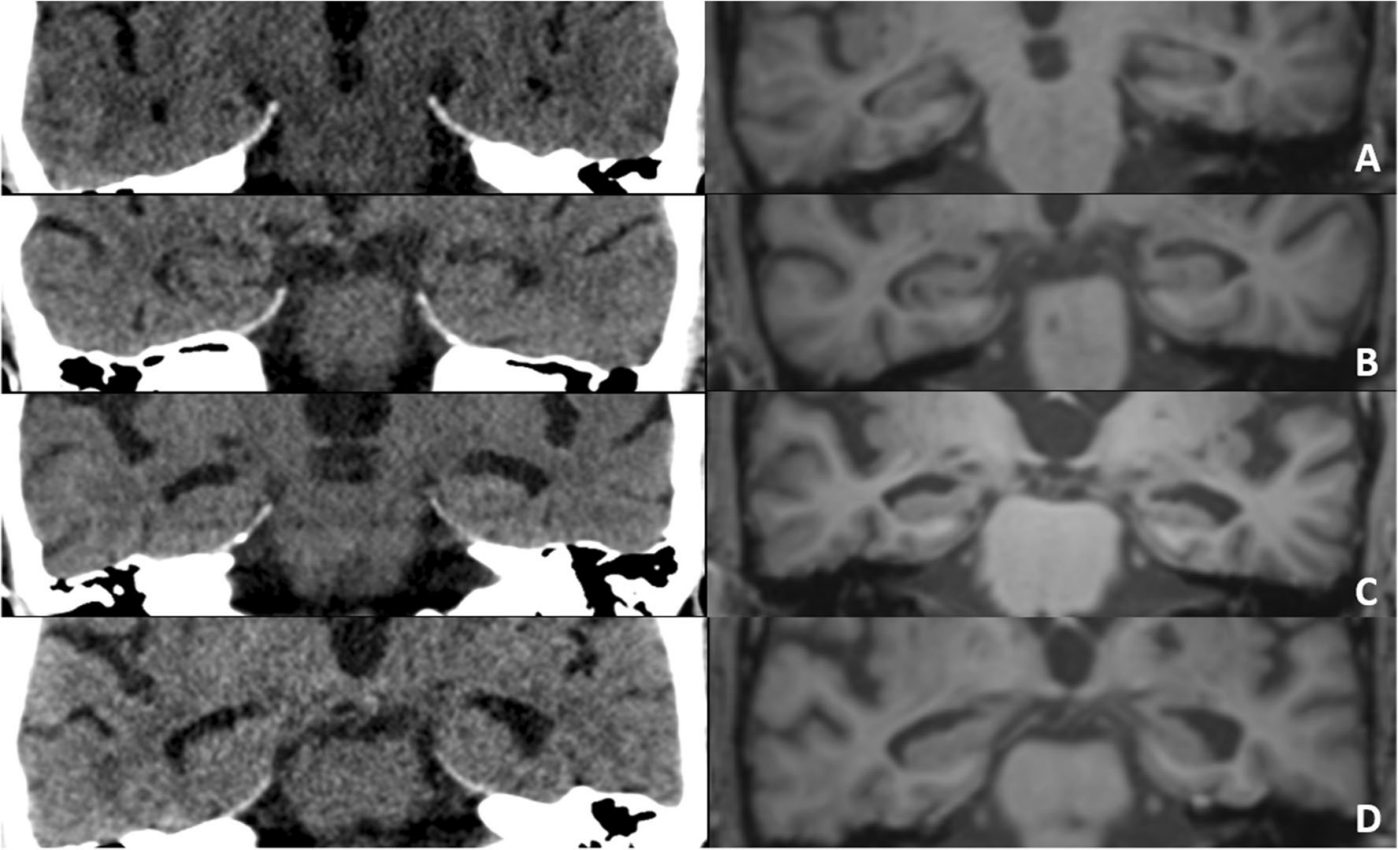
Image examples (CT to the left) of rater discrepancies for four subjects. Each side was rated separately, and the overall highest score was given. Subject “A”: MTA was graded “0” by two raters and graded “1” by two raters on both CT and MRI. Subject “B”: MTA was graded “1” by two raters and “2” by two raters on CT; MTA was graded “1” by one rater and “2” by three raters on MRI. Subject “C”: MTA was graded “2” by two raters and “3” by two raters on CT; MTA was graded “3” by all raters on MRI. Subject “D”: MTA was graded “2” by one rater and “3” by three raters on CT; MTA was graded “3” by three raters and “4” by one rater on MRI. Abbreviations: MTA, medial temporal lobe atrophy
